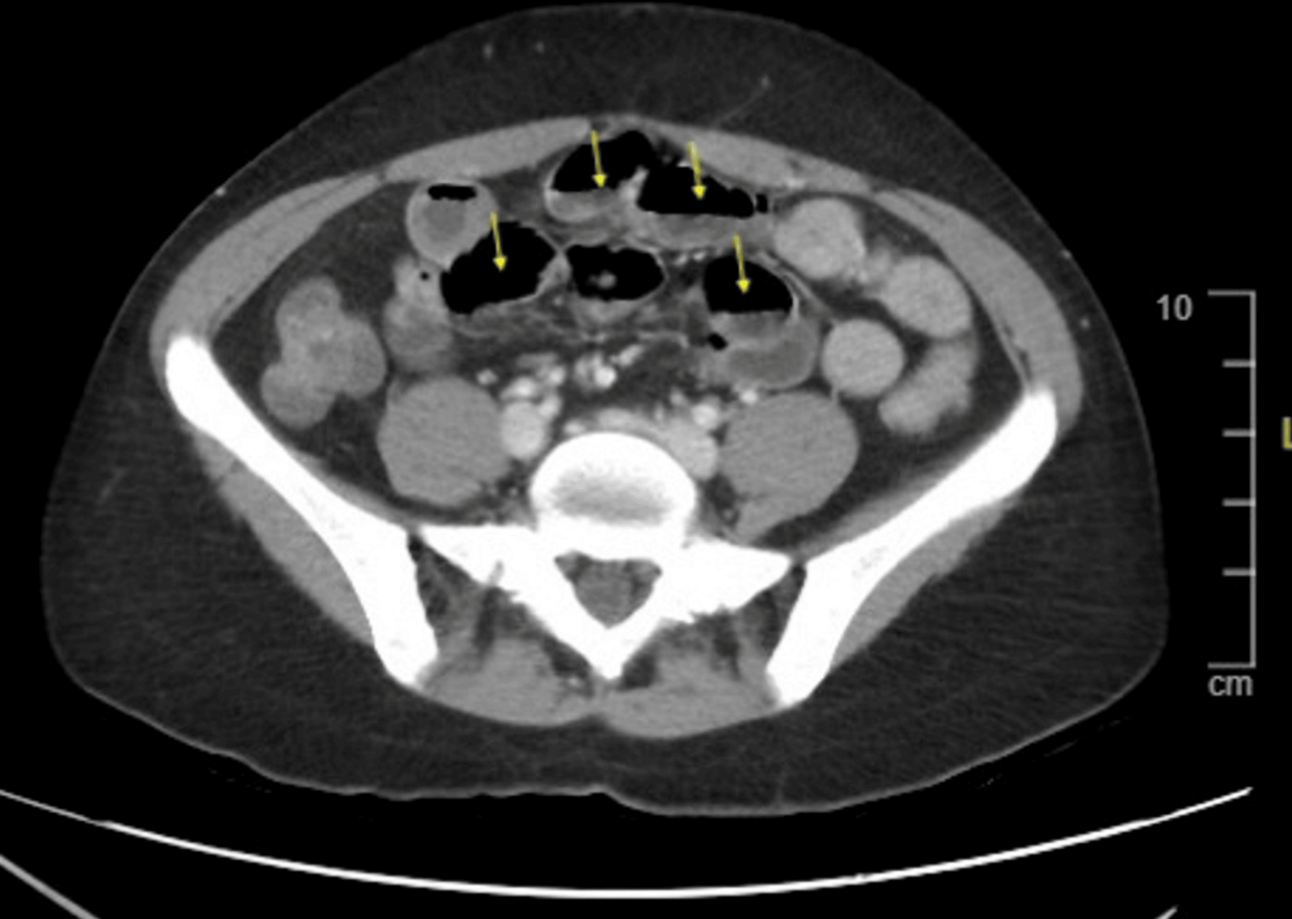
Axial view abdominal CT scan demonstrating fluid and air-filled loops of bowel denoted by yellow arrows.
